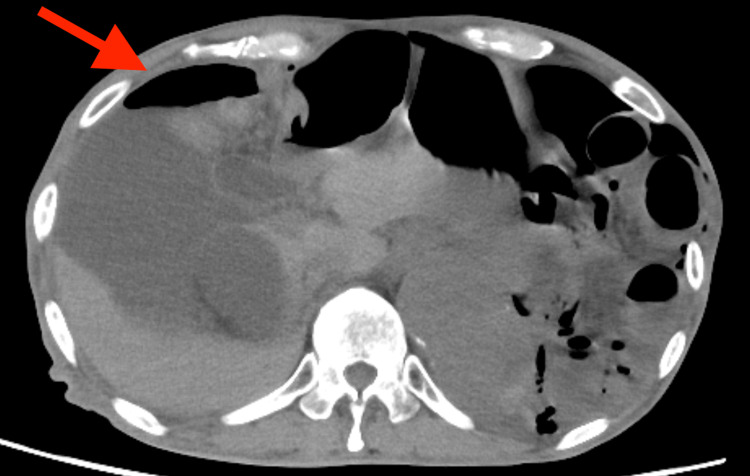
Abdominal computed tomography showing intra-abdominal free air
